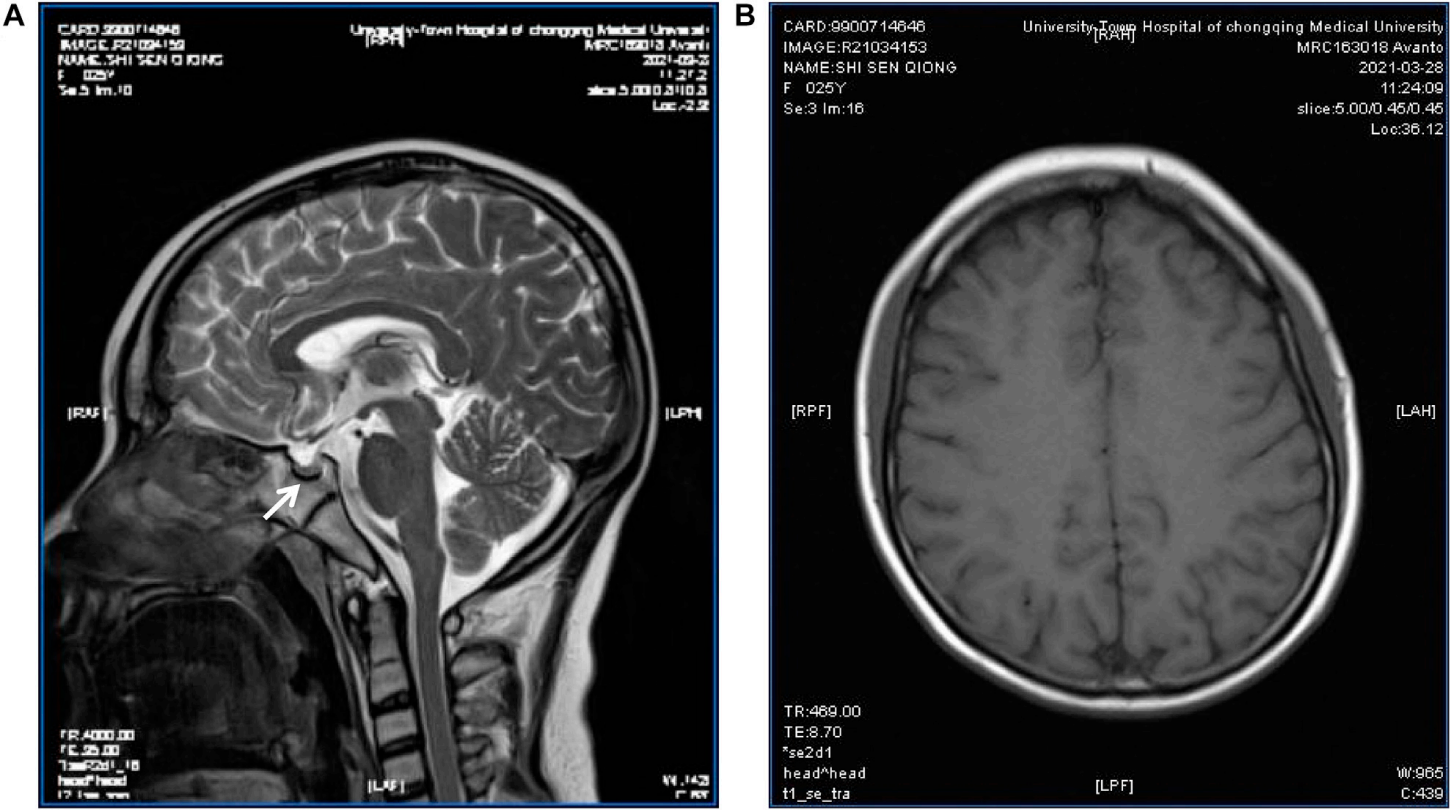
Pituitary MRI of the patient. T2-weighted MR imaging for the patient showed a partially empty sella and a small pituitary gland (arrow) (A); Magnetic sensitivity weighted imaging showed no abnormal iron deposition accumulation (B).
